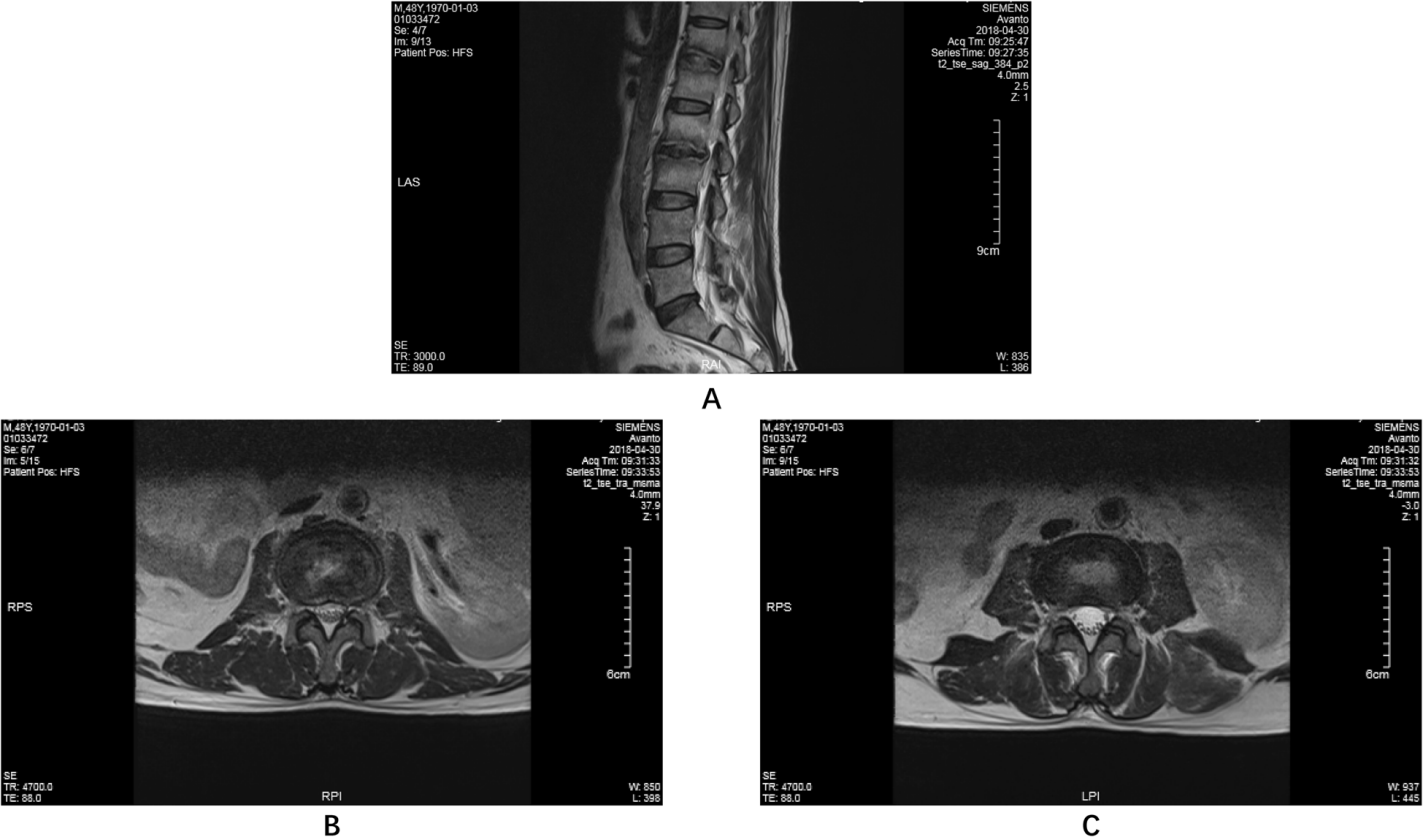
Lumbar MRI (2018-04-30) a sagittal plane. b transverse section of T12/L1 c transverse section of L2/L3
